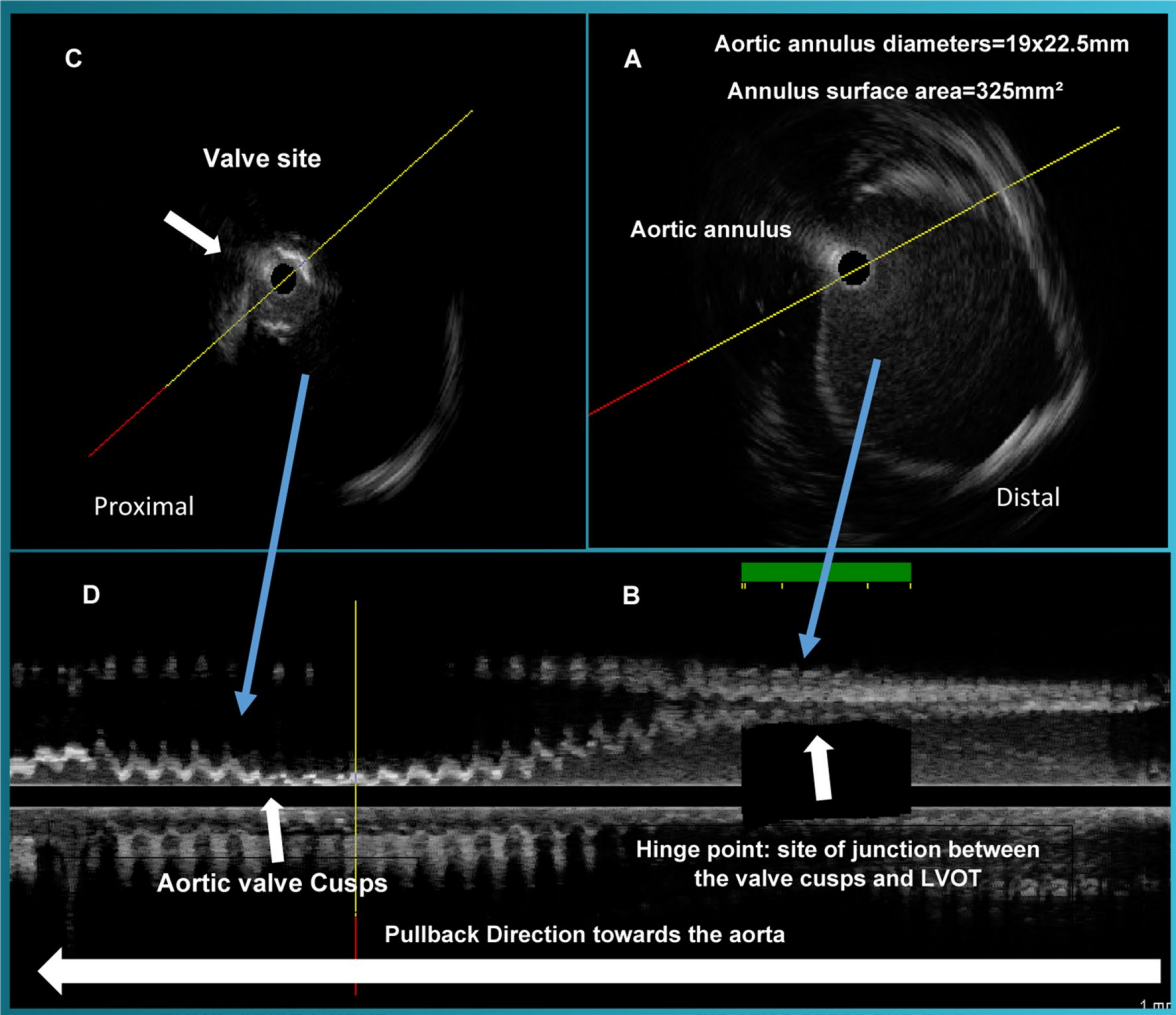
Intravascular ultrasound measurements of the aortic annulus diameters and surface area. The pullback is shown from right to left. A The IVUS image of the aortic annulus, the annulus area was 325 mm^2^; B corresponding longitudinal IVUS image at the site of junction between LVOT and the aortic cusps; C calcified aortic valve with signal drop-out; D corresponding longitudinal IVUS image of aortic valve cusps
